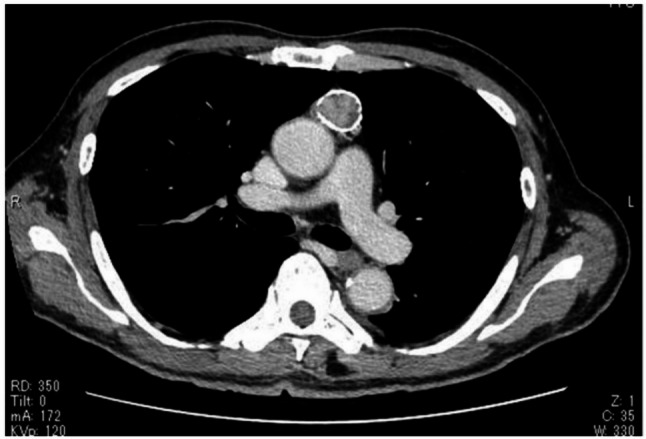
Chest computed tomography shows a 22-mm anterior mediastinal tumor with ring-shaped calcification
